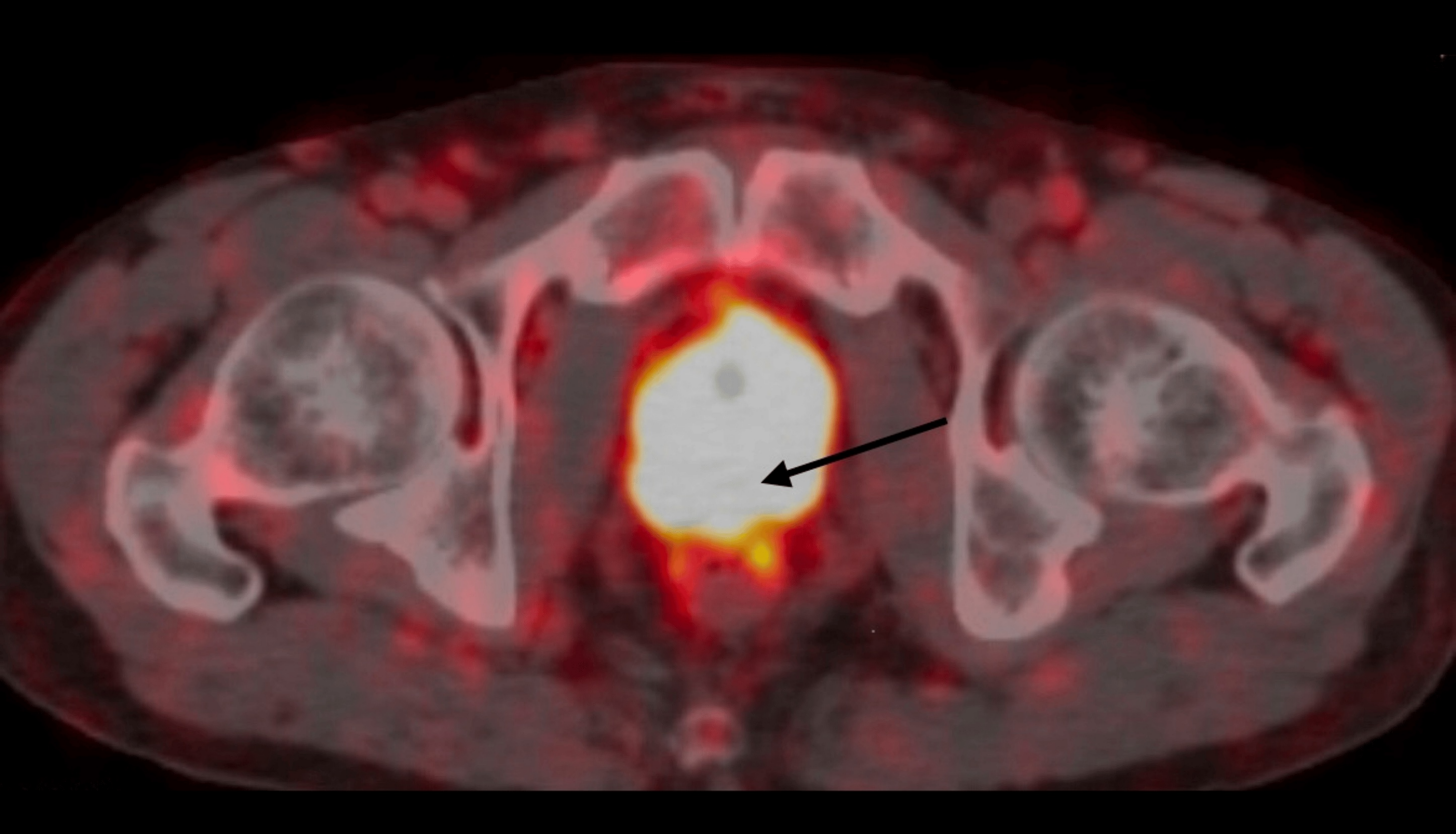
Ga-68 PSMA PET/CT revealing seminal vesicle infiltration by prostate cancer Ga-68 PSMA PET/CT imaging reveals heterogeneous tumor lesions infiltrating both seminal vesicles. The findings indicate advanced local disease with increased radiotracer uptake characteristic of prostate malignancy. CT, Computed tomography; PET, Positron emission tomography; PSMA, Prostate-specific membrane antigen
